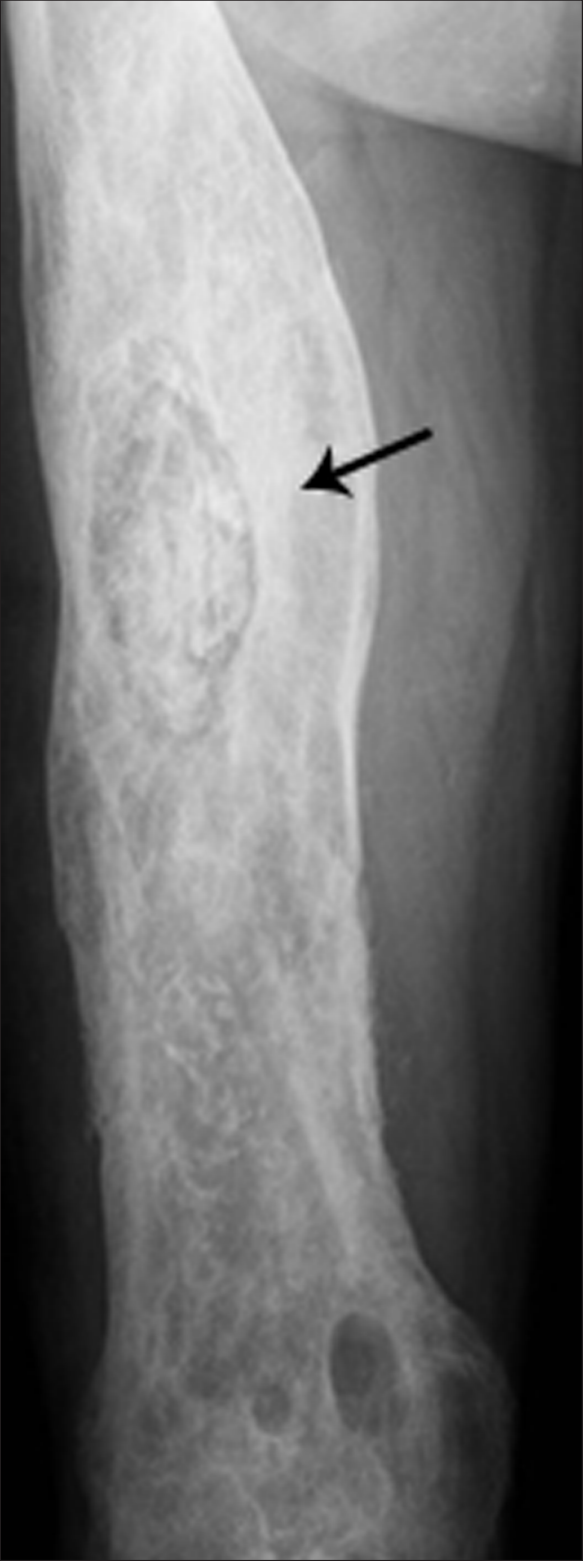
Chronic osteomyelitis on plain radiograph of the femur. Plain radiograph shows diffuse inhomogeneous osteosclerosis of the right femur with a focal area of increased opacity representing necrotic bone or sequestrum (black arrow). Plain films are often difficult to interpret because of superposition of viable and necrotic bone each with a different radiopacity.
